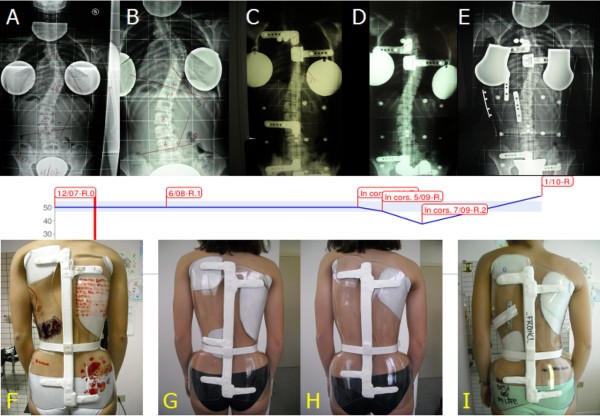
The Cheneauisation of the Sforzesco brace. We used the term Cheneauisation to underline that it derives from the contamination of our own brace with the internationally well-known Cheneau brace: in fact it aims at posturally changing the scoliosis curve through a thrust at level of the convexity of the apical proximal curve and an elevation/medialization of the shoulder at the concave side. Due to our own SPoRT principles the Cheneuization also includes an anteposition of this same shoulder. First line, from left to right: (A) x-rays of the patient at start of treatment (12/07), (B) after 6 months (6/08), (C) in-brace without Cheneuization (4/09); (D) in-brace with Cheneuization (5/09), (E) in-brace with Cheneuization after two months of treatment (7/09). Second line: graph of x-rays measurements. Third line, from left to right: (F) the first brace used; the brace trial: (G) without Cheneuization; (H) with Cheneuization; (I) the brace with Cheneuization at time of the 7/09 x-ray. This is the first situation in which we used the Cheneuization due to the absence of correction in a patient with an high-degree scoliosis refusing surgery, and presenting with a curve possibly responding to such a change. We then made two braces and compared their results with in-brace x-rays, with favorable results for the Chenuization (5/09), that was even increase by time and brace corrections (7/09). The final out-of-brace progression of scoliosis (1/10) was due to a sudden decrease on brace usage that this specific patient suffered.
